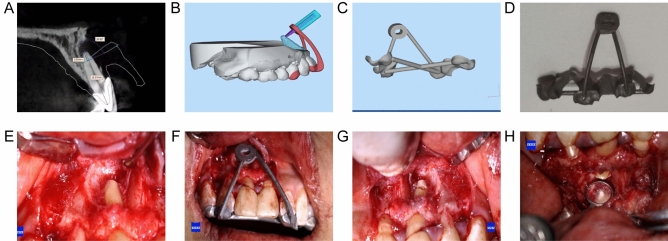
The design process and clinical application in endodontic microsurgery. (A) CBCT data of and the virtual bur data were merged in the designning software. (B) Establish the long axis of the target tooth and build the virtual bur-cutting trajectory. (C) Virtual model of 3D printed surgical guide. (D) Material object of 3D printed surgical guide. (E) The full-thickness mucoperiosteal flap was raised and the root apex and cyst wall were exposed. (F) The guide was aligned in position. (G) Root apex was extracted under the guidance of the 3D printed guide. (H) iRoot BP was backfilled into the cavity after bleeding control.
